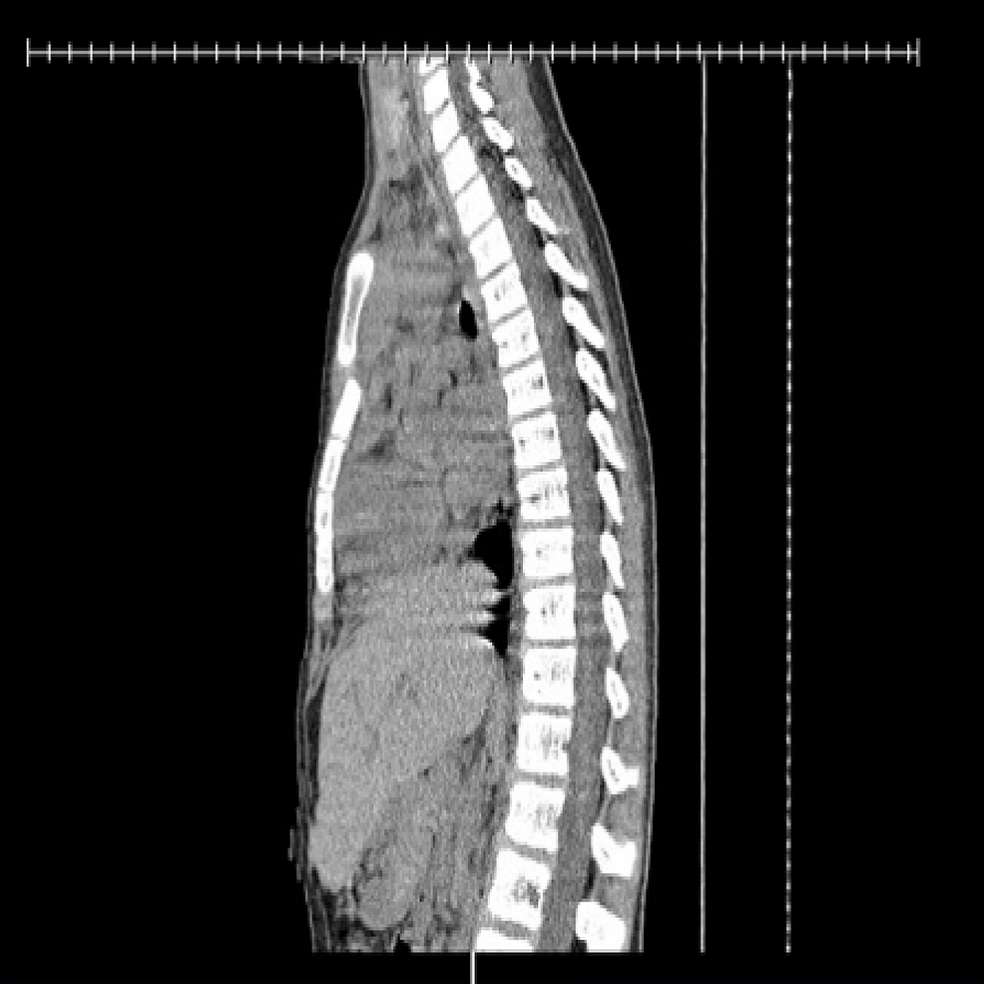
CT scan of the chest Computed tomography scan of the chest, lateral view demonstrating the posterior dislocation of the upper sternal body with respect to the manubrium. The dislocated upper sternal segment is approximately a whole sternal thickness posterior to the manubrium.
